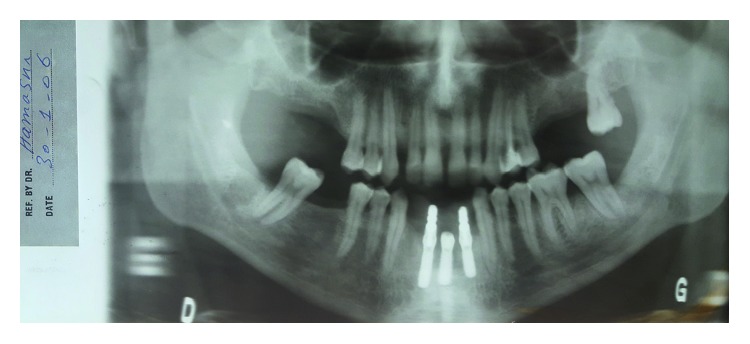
Panoramic X-ray after 3 months of first visit showing bone formation at the site of extracted molars and bone stability around Straumann mandibular implants.
